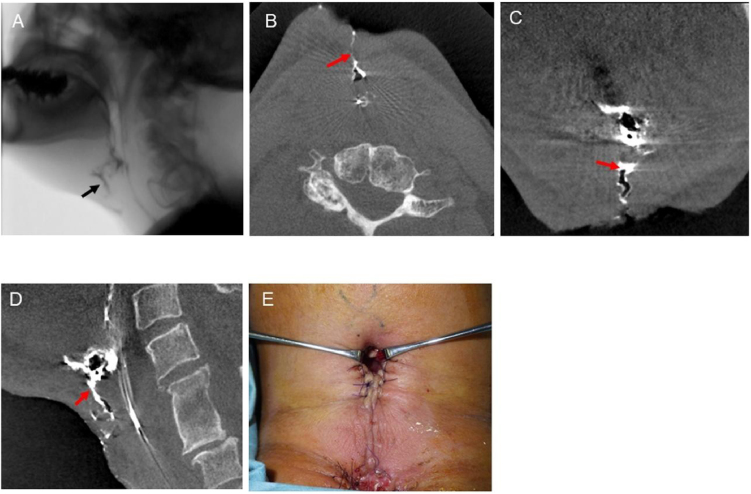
Videofluoroscopy and contrast CBCT findings of Case 1. A, Videofluoroscopy showing the leakage of contrast fluid. The black arrow indicates the leakage. (B–D) Axial, coronal, and sagittal images of contrast CBCT showing the accurate location of the contrast fluid. The red arrows indicate the leakage. E, Surgical treatment of pharyngocutaneous fistula was performed.
